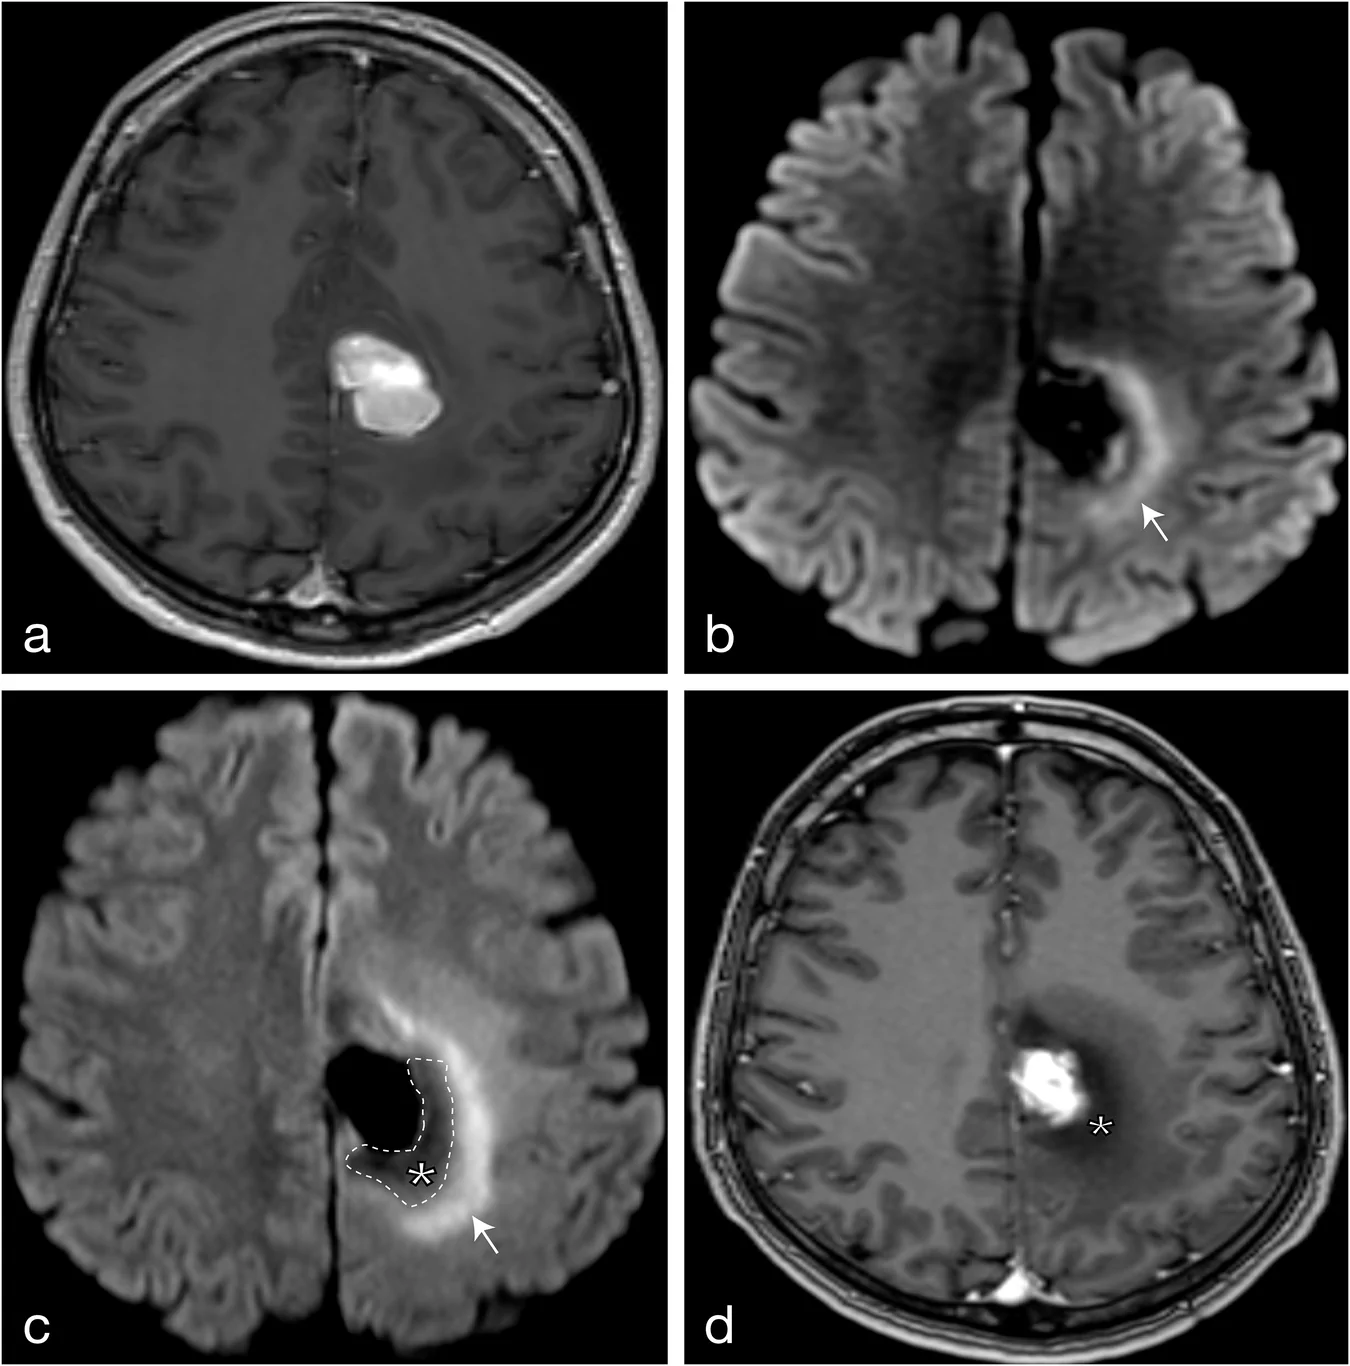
Radiation-induced white matter injury after stereotactic radiosurgery (SRS) for a parafalcine brain metastasis. a Pre-SRS axial post-contrast T1-weighted image showing the target metastasis. b Axial diffusion-weighted image at 12 months after SRS demonstrating a curvilinear band of high diffusion signal in the adjacent white matter (arrow), suggestive of demyelination. c Axial diffusion-weighted image at 32 months after SRS showing two co-existing zones: a peripheral band of high diffusion signal with directional progression along the white matter compared to panel b (arrow), suggestive of demyelination, and a central zone of low diffusion signal (dashed outline and asterisk), likely representing established gliosis. d Axial T1-weighted image at 32 months showing T1 hypointensity at the same site as the low-DWI zone in c (asterisk), in keeping with chronic gliosis
